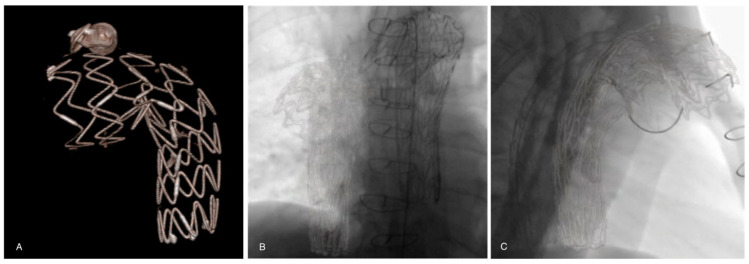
(A) A previous thoracic stent-graft three-dimensional volume rendering model reconstructed from the preoperative CTA. Registration and overlay are performed with two fluoroscopic orthogonal antero-posterior (B) and lateral (C) shots.
